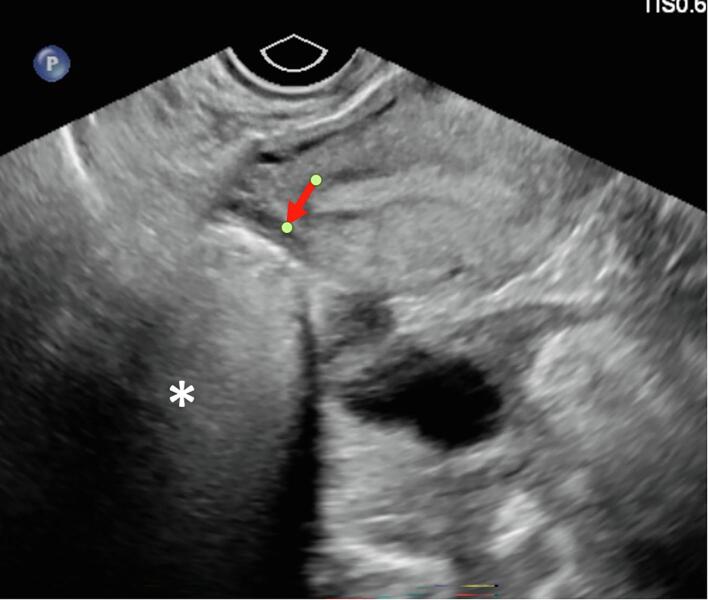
26-year-old female with lower abdominal pain, on ultrasound had bilateral mature teratomas measuring approximately 4 cm. Transvaginal ultrasound demonstrates hyperechoic mass (red arrow) with posterior attenuation (white *), known as the tip of the iceberg sign. Posterior and superior to the uterus fundus, solid mass with cystic component is partially visualized, demonstrating large pelvic mass with mixed solid and cystic component with minimal scattered fat better identified on CT.
